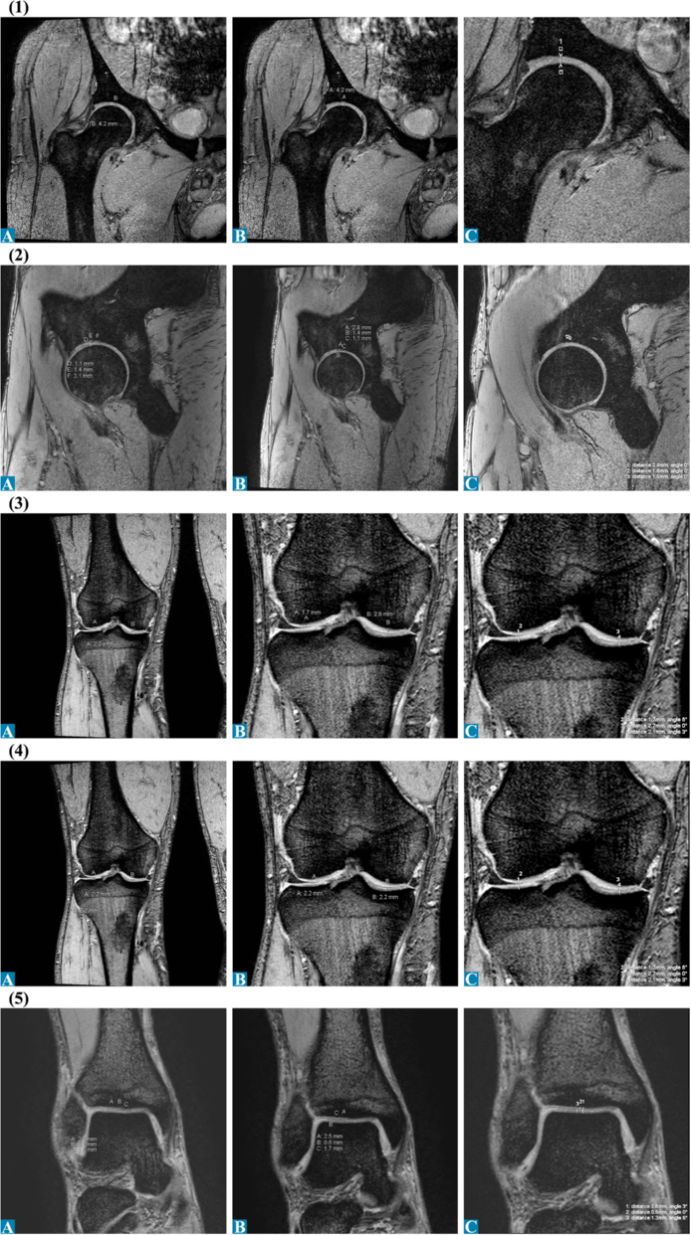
Articular cartilage thickness measurements of the right leg of radiologists A-C for 3.0 T MRI: (1) Hip coronal; (2) Hip sagittal; (3) Femur coronal; (4) Tibia coronal; (5) UAJ coronal.
